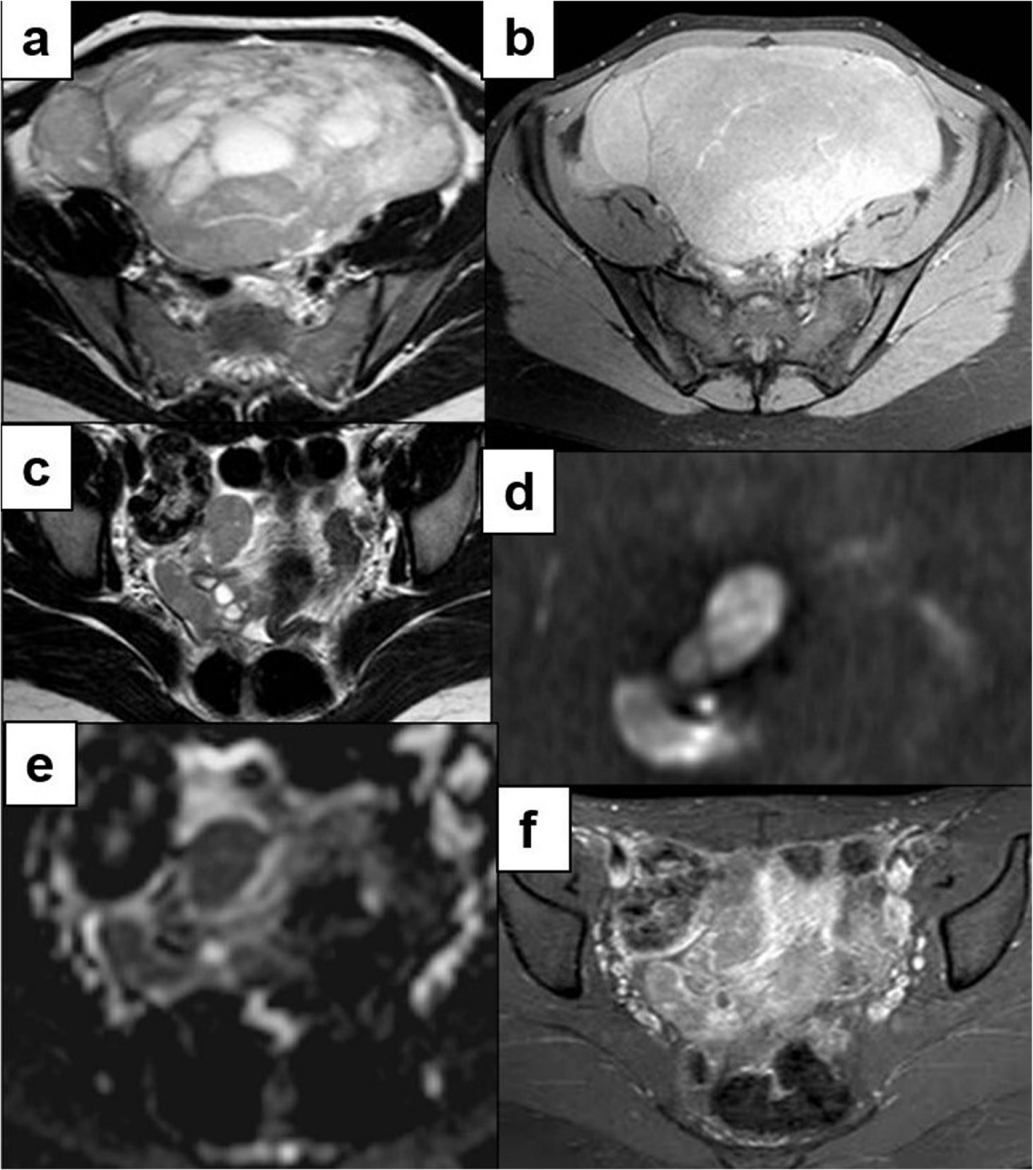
Ovarian dysgerminoma in a 23-year-old woman with recurrence after surgery. Axial T2w (a), axial contrast-enhanced T1w with fat-suppression (b) MR images show a multilobulated heterogeneous large-sized cystic-solid pelvic mass with interspersed septa and avid enhancement after Gd administration. The mass was surgically removed and stage II dysgerminoma was diagnosed. Two years after surgical treatment, during a routine follow-up, we revealed a recurrence in the right emipelvis, characterized by heterogeneous hyperintense signal on axial T2w (c), high signal and low values on DWI and ADC images (d, e), heterogenous moderate enhancement after Gd administration (f)
